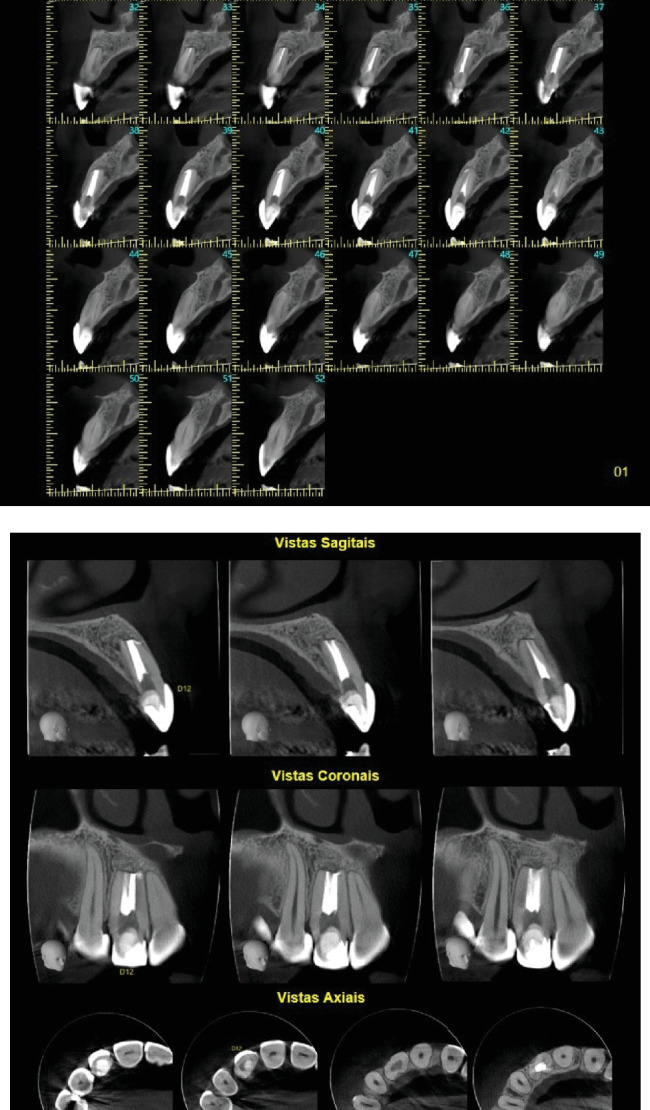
(a, b) Cone-beam computed tomography with small volume and high resolution, with axial, sagittal, coronal, and parasagittal cuts of 1-mm thickness and spacing of 0.5 mm at 0.5-mm intervals. Tooth 12 with satisfactory endodontic treatment shows a dental anomaly in shape. There is an observed flattening of the root apex, consistent with periapical surgery. The structure of the remaining alveolar bone tissue is normal, as is the density of the spongy bone.
